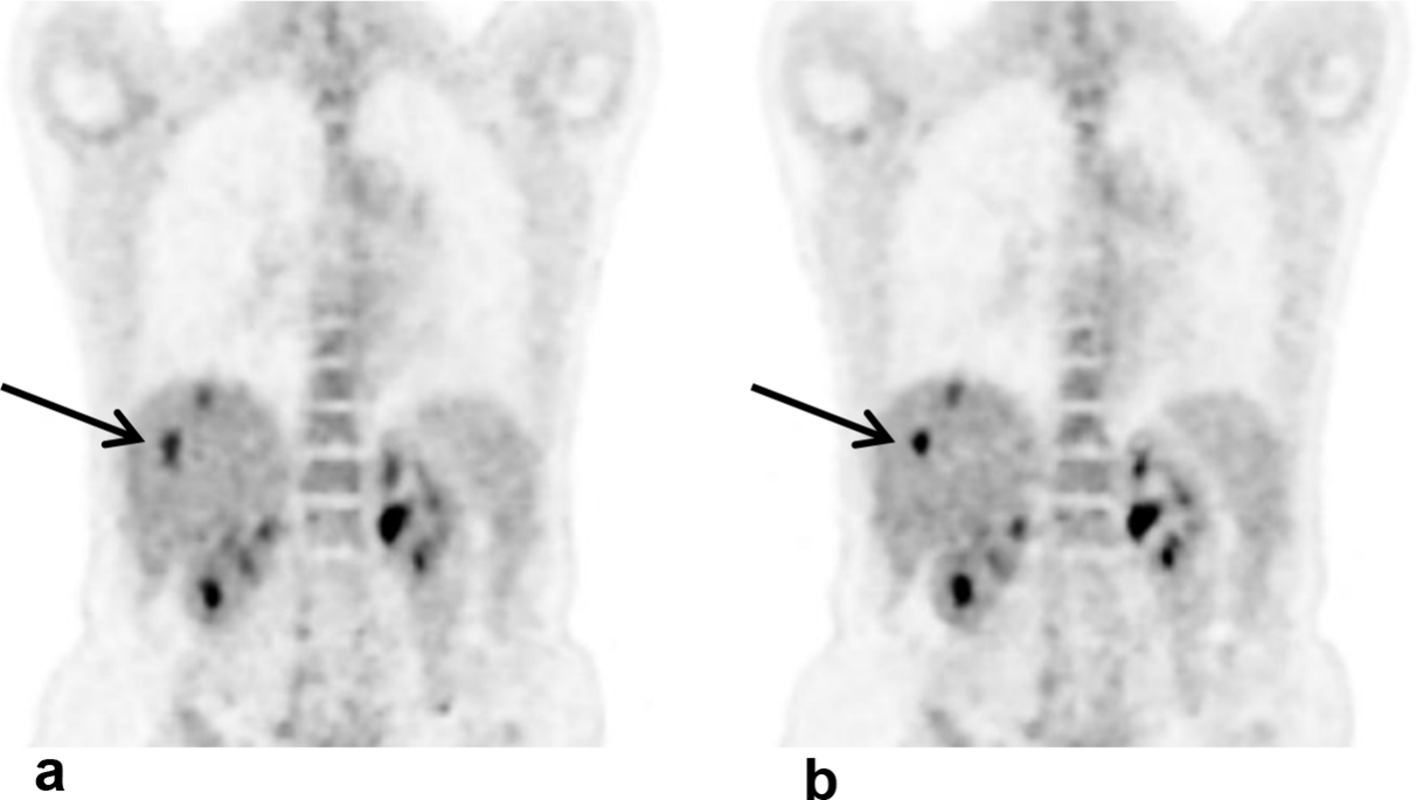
Example images from a patient demonstrating the improved contrast and visual image quality that can be obtained using respiratory gating. A coronal slice is shown. (a) Without motion compensation (using 2 min of data). (b) With data-driven quiescent period gating (using 4 min of data, reduced to approximately 2 min after gating). The linear grey scale is from an SUV of 0–6. SUV, Standard uptake value.
